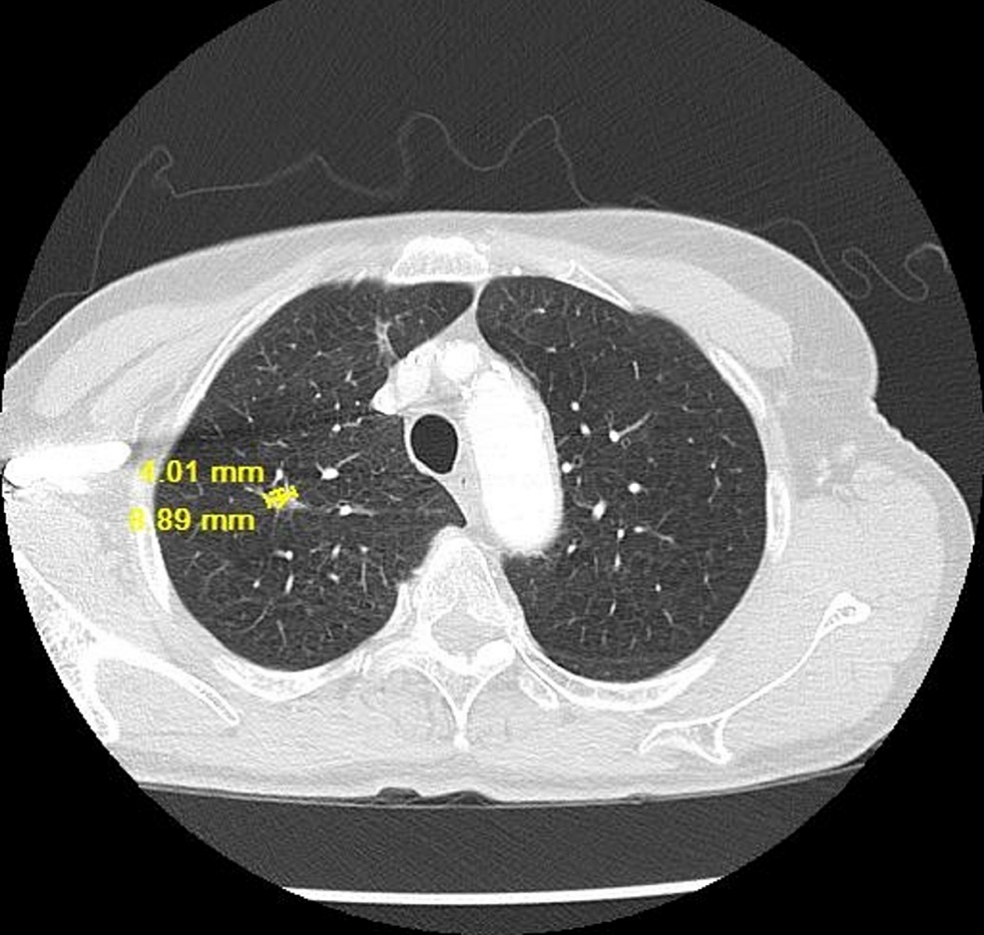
CT chest lung window showing resolving lung nodule on 10/26/2018
